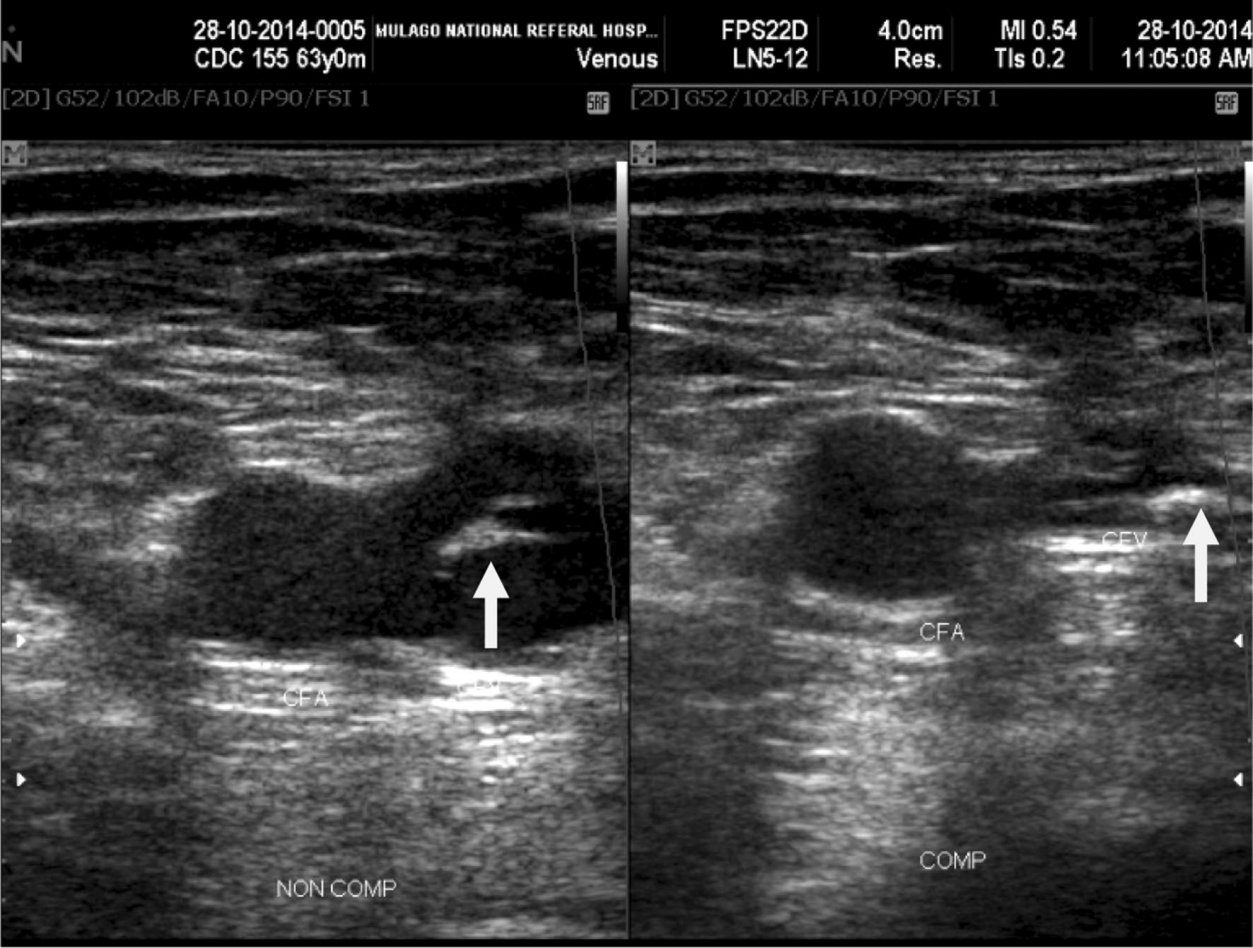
Chronic DVT. Transverse sonogram of 63 years old male HIV positive patient on HAART shows echogenic thrombus (white arrow) in the right common femoral vein (CFV) causing partial compressibility of the vein
